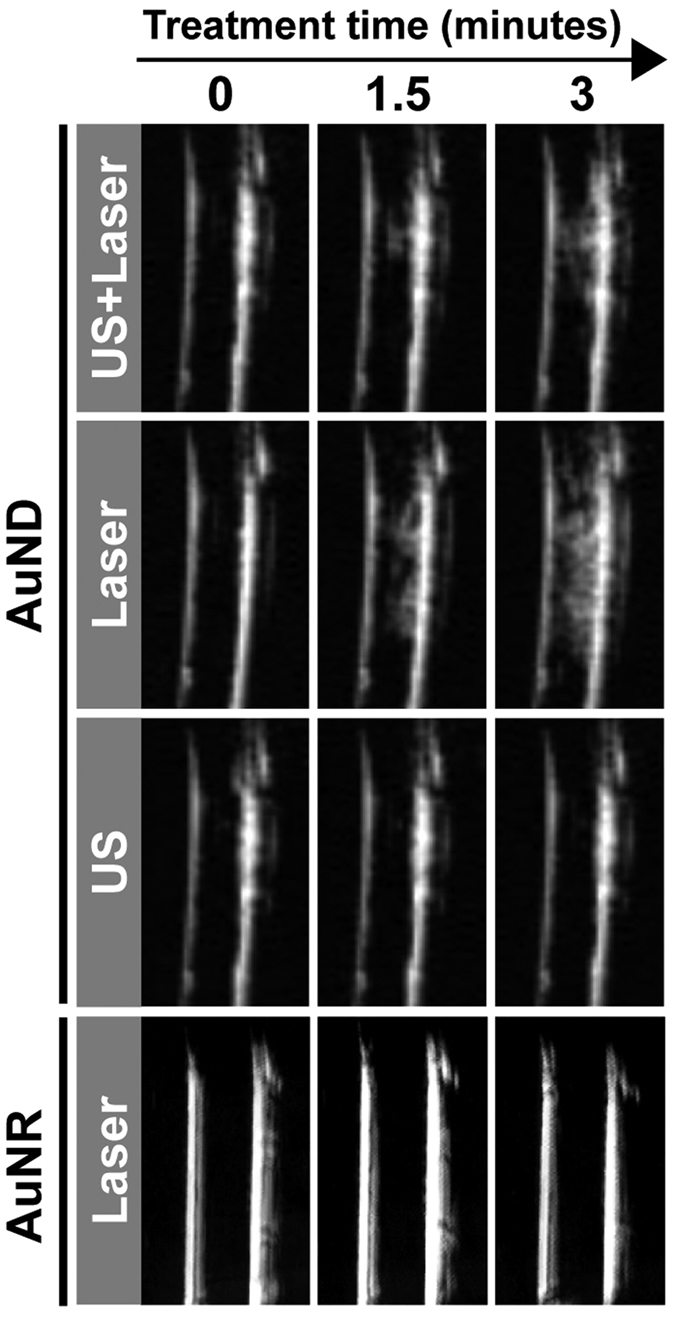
Vaporization of AuNDs. B-mode images showing the temporal profile of the vaporization of AuNDs during exposure to ultrasound only, laser radiation only, ultrasound plus laser radiation, or AuNRs exposed to laser radiation only at 0 minutes, 1.5 minutes, and 3 minutes (left, middle, and right panels). The two white near-vertical linear areas in each image correspond to the walls of the tubes.
